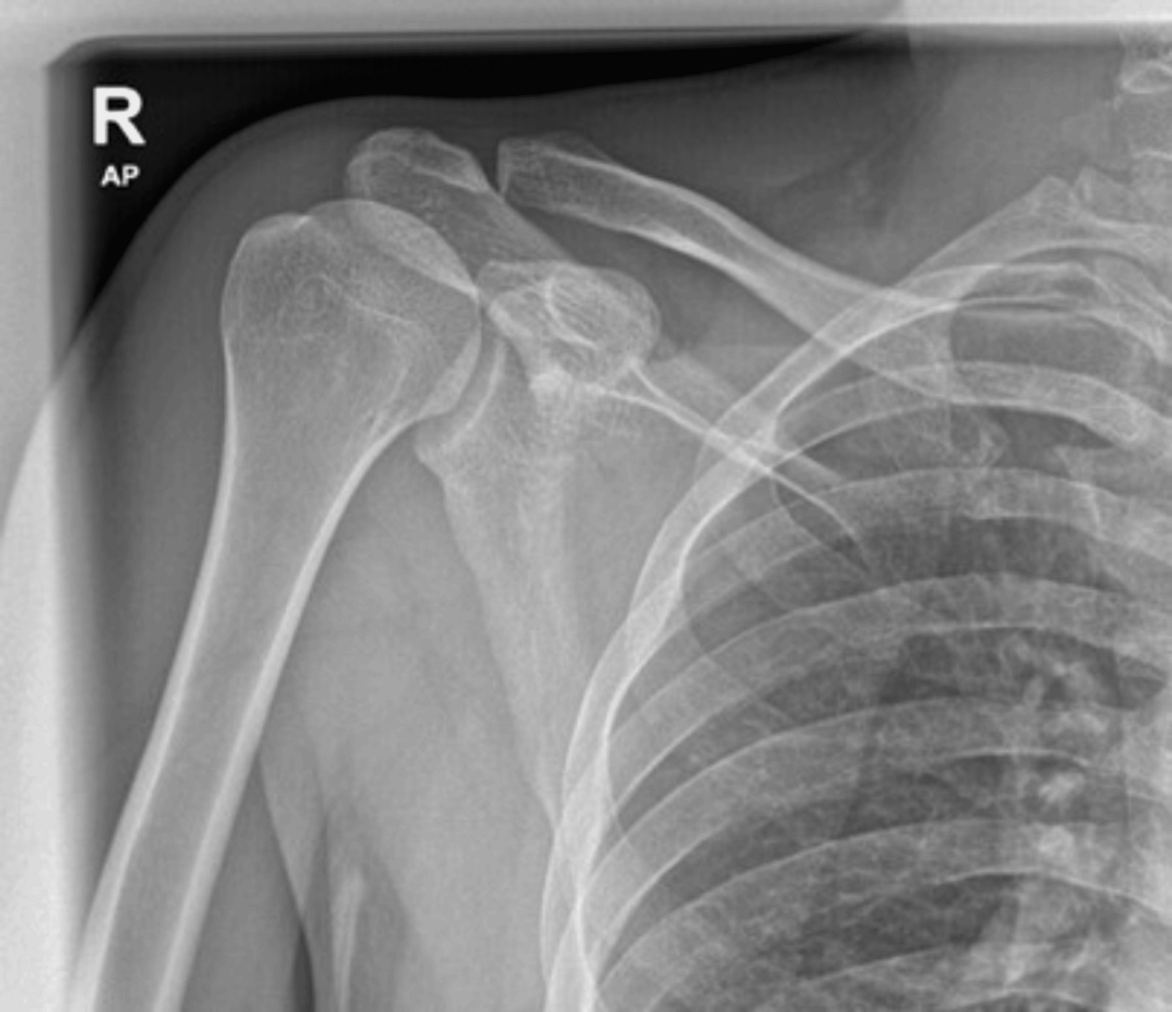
Right shoulder X-ray revealed unremarkable findings.
